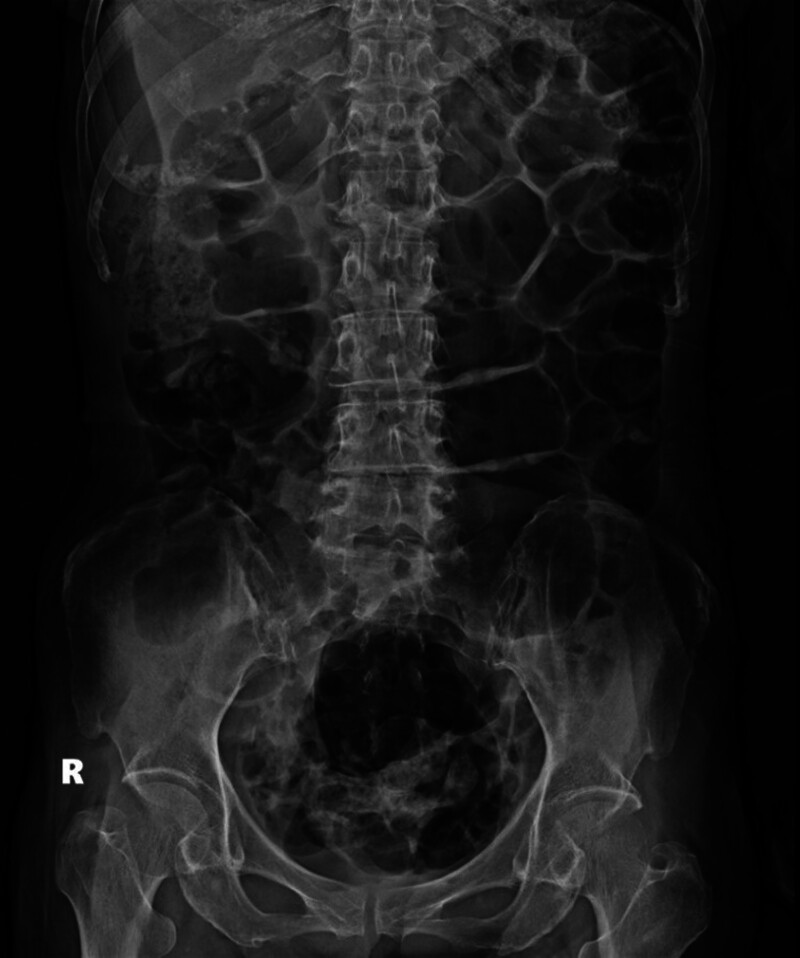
Kidney, ureter, and bladder X-ray of this patient.
